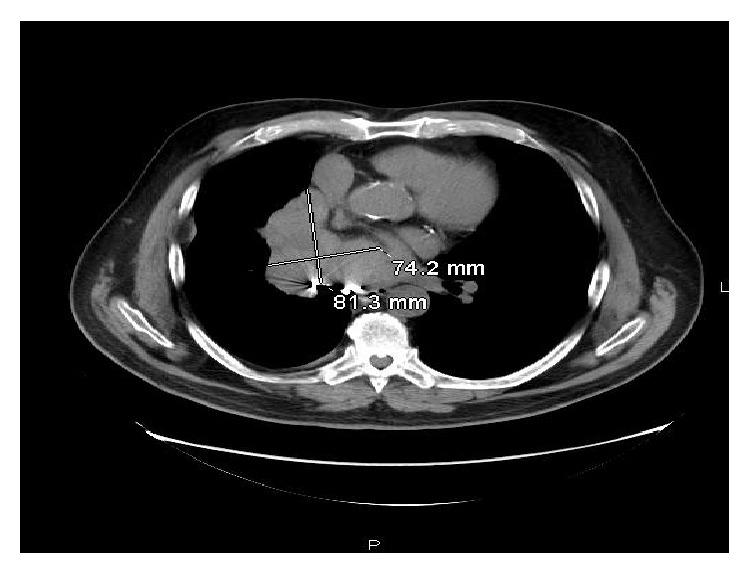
CT Scan of chest showing right hilar mass.
